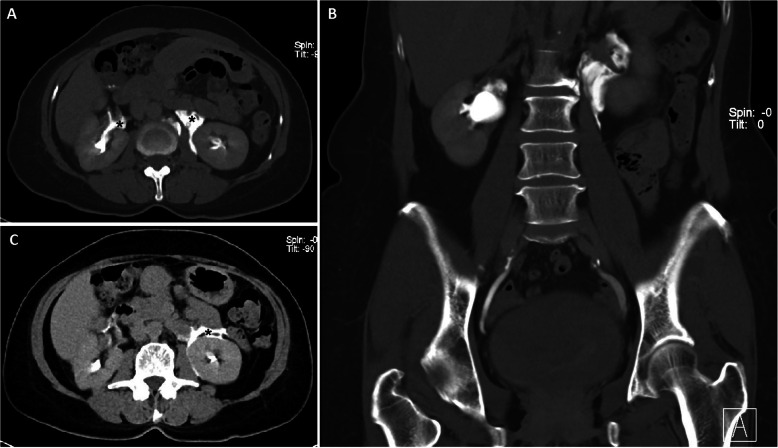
Initial delayed phase of the CT urogram (a, b) with peripelvic contrast extravasation marked with asterisks. Further delayed phase performed at 50 min (c) showing tracking of contrast
